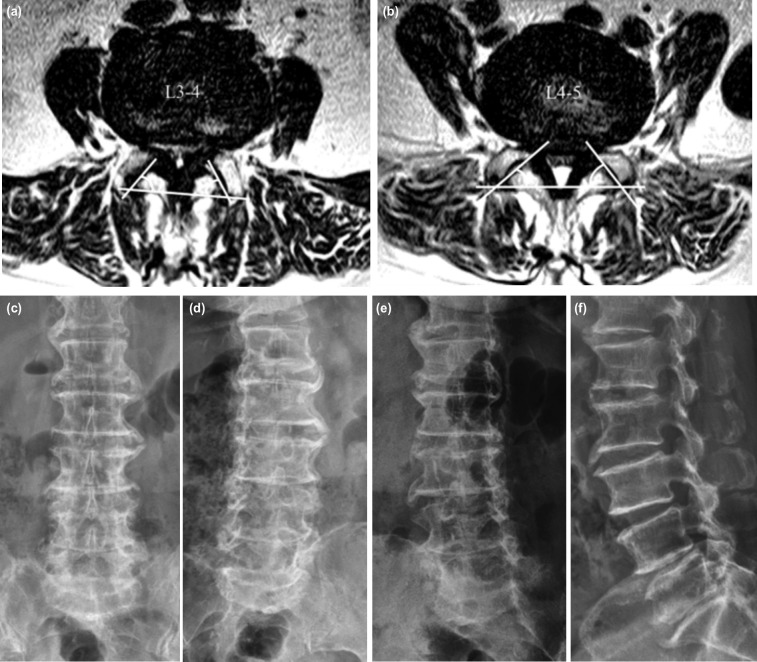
Pre-operative MRI and radiograph image. (a) L3-4 transverse and (b) L4-5 transverse MRI T2 weighted image shows moderate to severe central canal stenosis, and facet tropism at L4-5 (mean axial facet angle of 49°; 38° in right facet, 60° in left facet). (c) Standing AP. (d) Left oblique. (e) Right oblique. (f) Lateral radiograph shows disc height had been decreased to 6mm.
